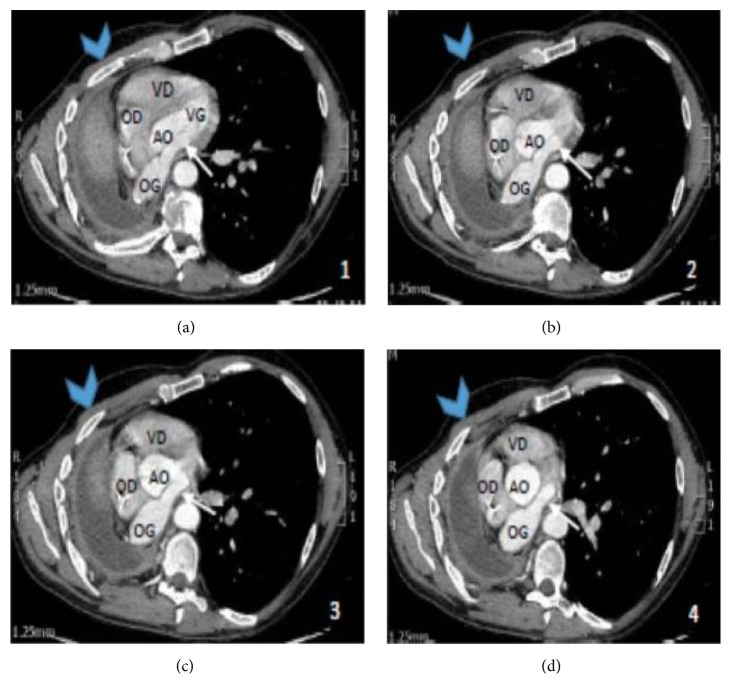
Thoracic computed tomography. VD, right ventricle; VG, left ventricle; OD, right atrium; OG, left atrium; AO, ascending aorta.
